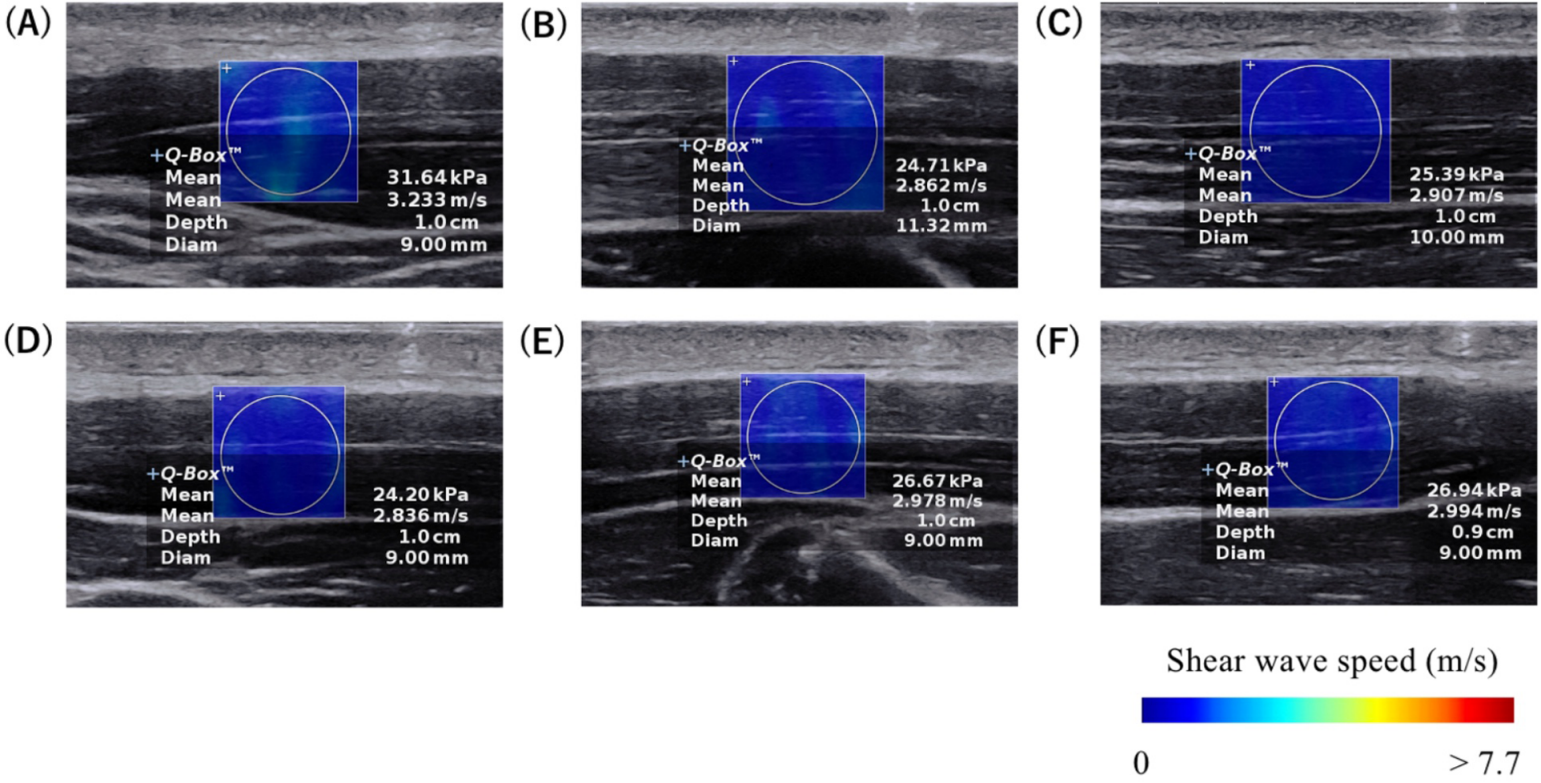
Typical examples of ultrasound SWE images at the (A) proximal, (B) central, and (C) distal sites of the anterior part, and at the (D) proximal, (E) central, and (F) distal sites of the posterior part of the upper trapezius. The colored region represents the shear wave speed map, with the scale shown at the bottom of the images.
